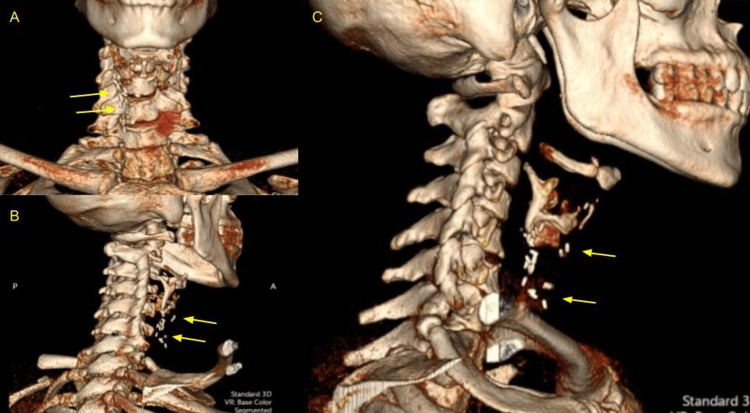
3D CT scan of the neck with frontal (A), posterolateral (B), and lateral (C) views showing surgical clips (yellow arrows).
